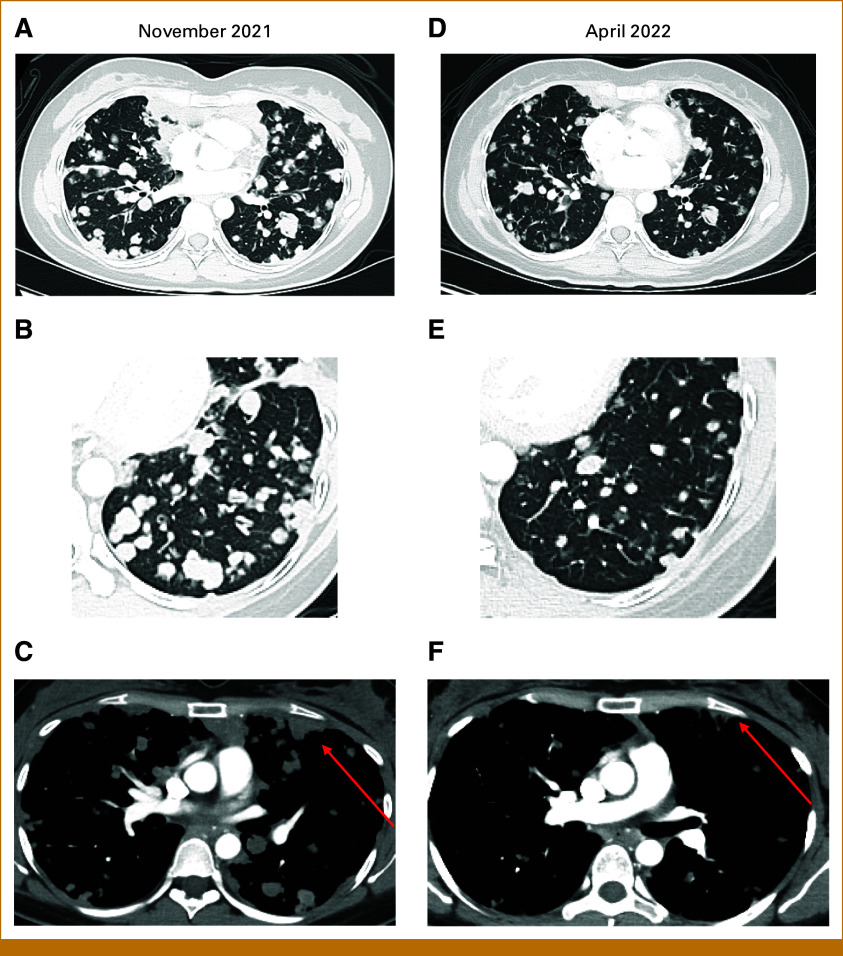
Chest CT showing response to treatment with AXL-CAB-ADC. Axial images with lung window show a reduction in the size and number of parenchymal metastatic nodules (A and B) from the baseline examination (November 2021) (D and E) to the on-treatment examination (April 2022). In addition, (C) chest CT with contrast (mediastinal window) reveals metastatic pleural disease (arrow) characterized by nodular pleural thickening in the baseline examination (November 2021). (F) Post-treatment examination (April 2022) reveals a significant decrease in the pleural lesions (arrow). CT, computed tomography.
